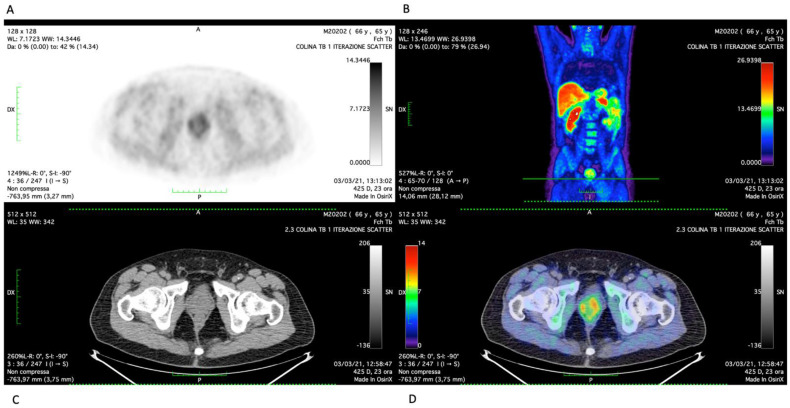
[^18^F]Choline positron emission PET-CT scan; (A) attenuation-corrected (AC) view; (B) maximum-intensity projection (MIP) view; (C) axial CT view; (D) axial fused view.
